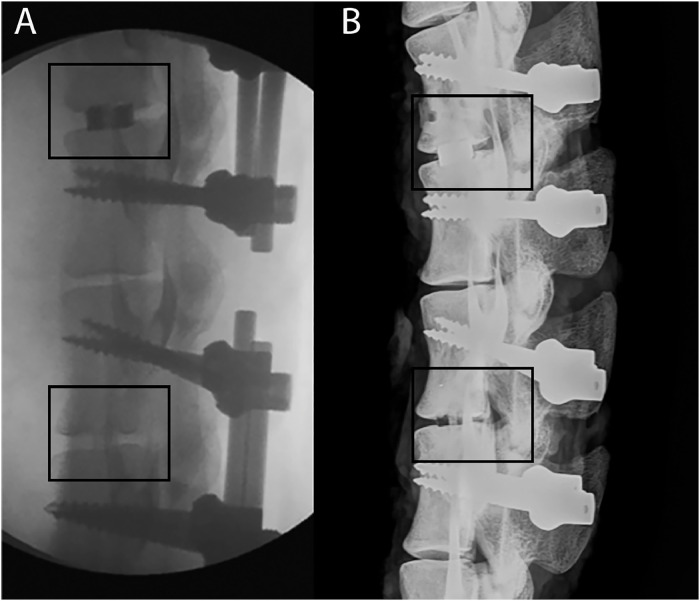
Lateral intra-operative fluoroscopy (A) and Faxitron radiograph (after the rods were removed) at 8 weeks (B). The top level (L23) was implanted with a 3DTi cage filled with autograft which can be visualised due to the radio-opacity of titanium. The bottom level (L45) was implanted with a 3DPEEK caged filled with autograft. The 3DPEEK cage cannot be detected while the graft at the time of surgery is noted as well as a bony bridge at 8 weeks.
